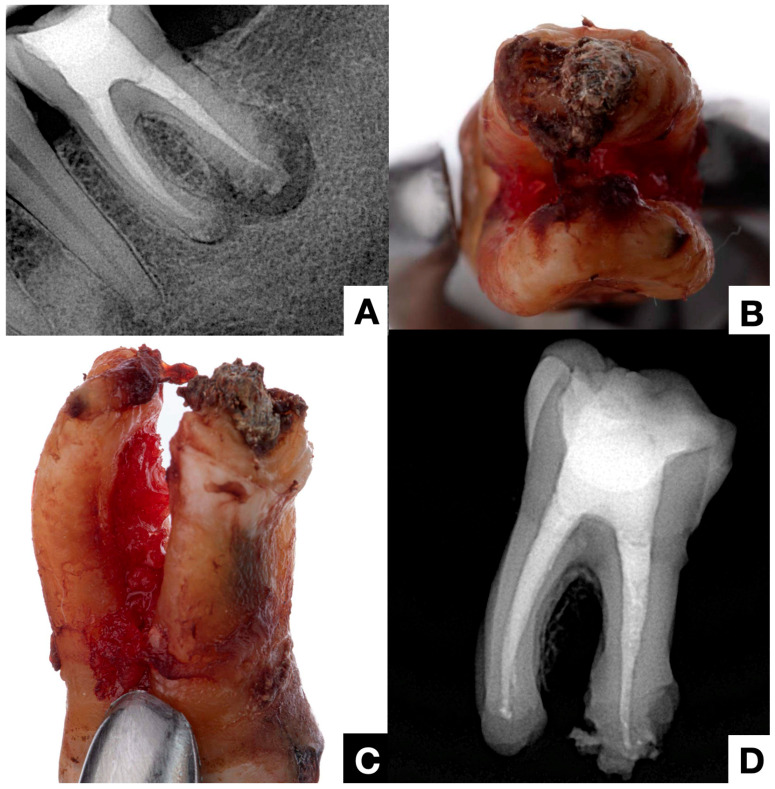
(A) Periapical radiograph of a previously treated tooth showing apical periodontitis, apical root resorption, and a radiopaque image on the distal root suggestive of apical calculus. Despite an adequate treatment, the patient experiences persistent pain, refuses further treatment, and opts for extraction. (B,C) Post-extraction images revealing extraradicular infection at the distal root apex. The tooth exhibited no periodontal probing. (D) Post-extraction radiograph showing more evident evidence of apical biofilm mineralization (clinical case courtesy of Javier Domínguez Bernal).
